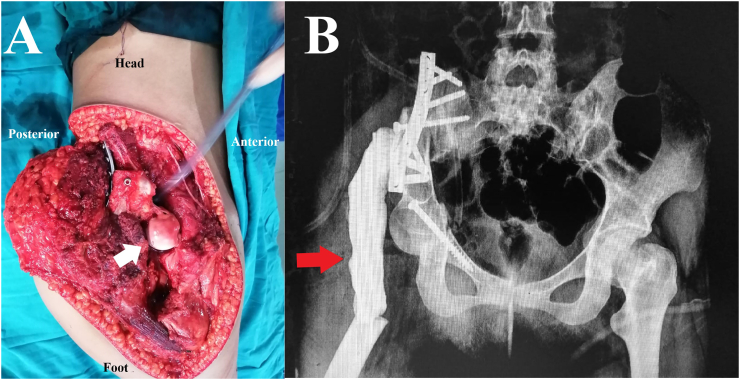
Surgical intervention, the first stage formed of, A: resection Type I and II according to the classification by Enneking and Dunham of the right hemipelvis and upshifting of the proximal femur to reconstruct the defect (white arrow pointing to the femoral head placed near the resected native acetabulum). B: immediate postoperative pelvis anteroposterior plain radiographic view showing the proximal femur upshifted and fixed to the pelvis and a cement spacer (red arrow) placed to compensate for the resected proximal femoral segment. (For interpretation of the references to colour in this figure legend, the reader is referred to the web version of this article.)
